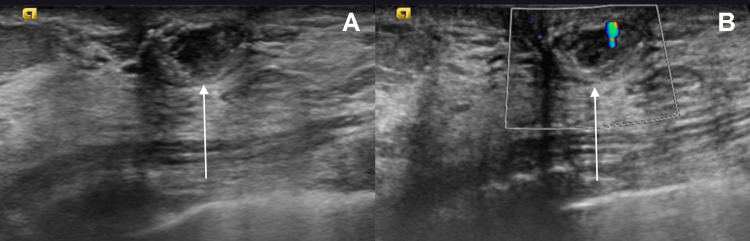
Ultrasonography of the left breast and axilla revealed a 10 x 10 x 6 mm solid mass with heterogeneous echotexture and coarse calcifications at 9 o’clock (A), 1 cm from the nipple. No internal color flow was appreciated on color Doppler imaging (B). The remainder of the breast and axilla ultrasound was unremarkable.
